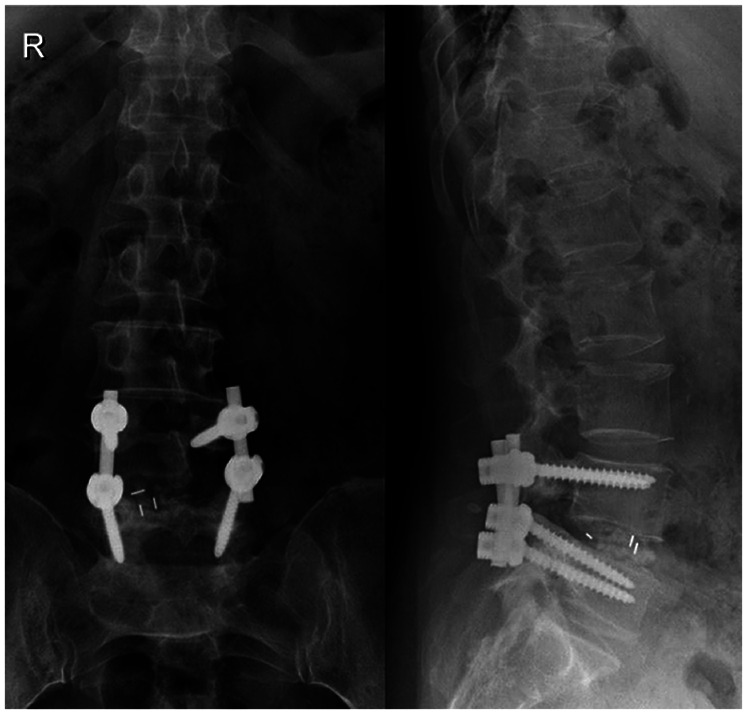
Postoperative lumbar anteroposterior and lateral radiographs.
